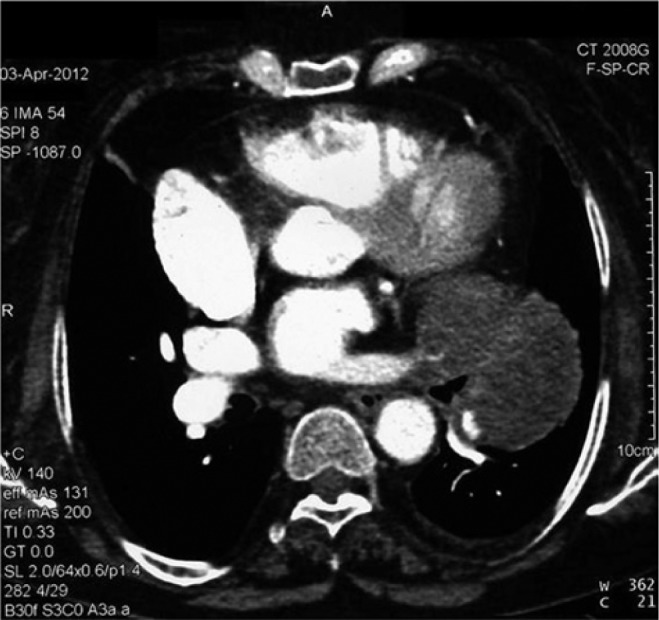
Chest spiral CT scan with contrast showing a large lobulated hypodense mass in the left
